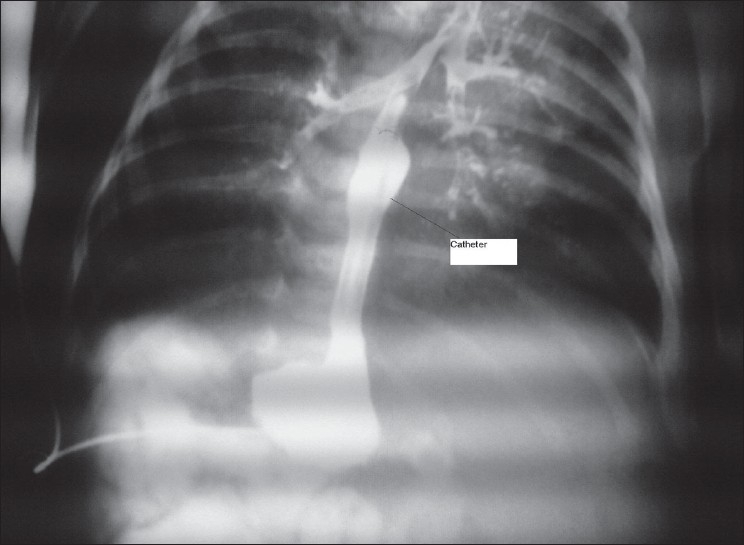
X-ray shows spillage of contrast material and tube entering bronchus
